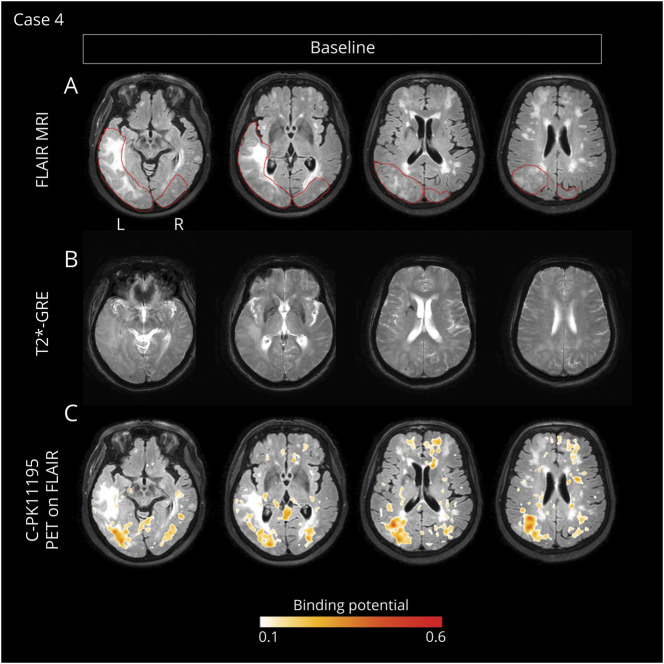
Longitudinal In Vivo Imaging of Microglial Activation During the Course of Spontaneous ARIA in a Patient With Possible CAA-ri (Case 4) Baseline MRIs and ^11^C-PK11195 PET acquired 3 weeks after symptom onset. (A) T2-weighted (FLAIR) images showed spontaneous ARIA-E in the left and right occipital lobes (red lines indicate the anatomic regions affected by ARIA-E). (B) Gradient echo-T2*-weighted imaging (GRE-T2*) sequence, coregistered to baseline FLAIR, showed disseminated cortical and subcortical microbleeds in the left temporal lobe and subarachnoid hemorrhage in the left parietal lobe. CSF testing confirmed high concentrations of anti-Aβ autoantibodies.^2^ A diagnosis of probable CAA-ri was made.^6^ (C) ^11^C-PK11195 PET binding potential peaks (BP peaks), coregistered and superimposed onto baseline FLAIR, reveal diffuse BP peak clusters of microglial activation that were mainly colocalized within the ARIA-E anatomic regions. The patient was treated with high-dose corticosteroid pulse therapy, with marked improvement of clinical symptoms. ARIA-E = amyloid-related imaging abnormalities suggestive of vasogenic edema; CAA-ri = cerebral amyloid angiopathy–related inflammation; FLAIR = fluid-attenuated inversion recovery.
